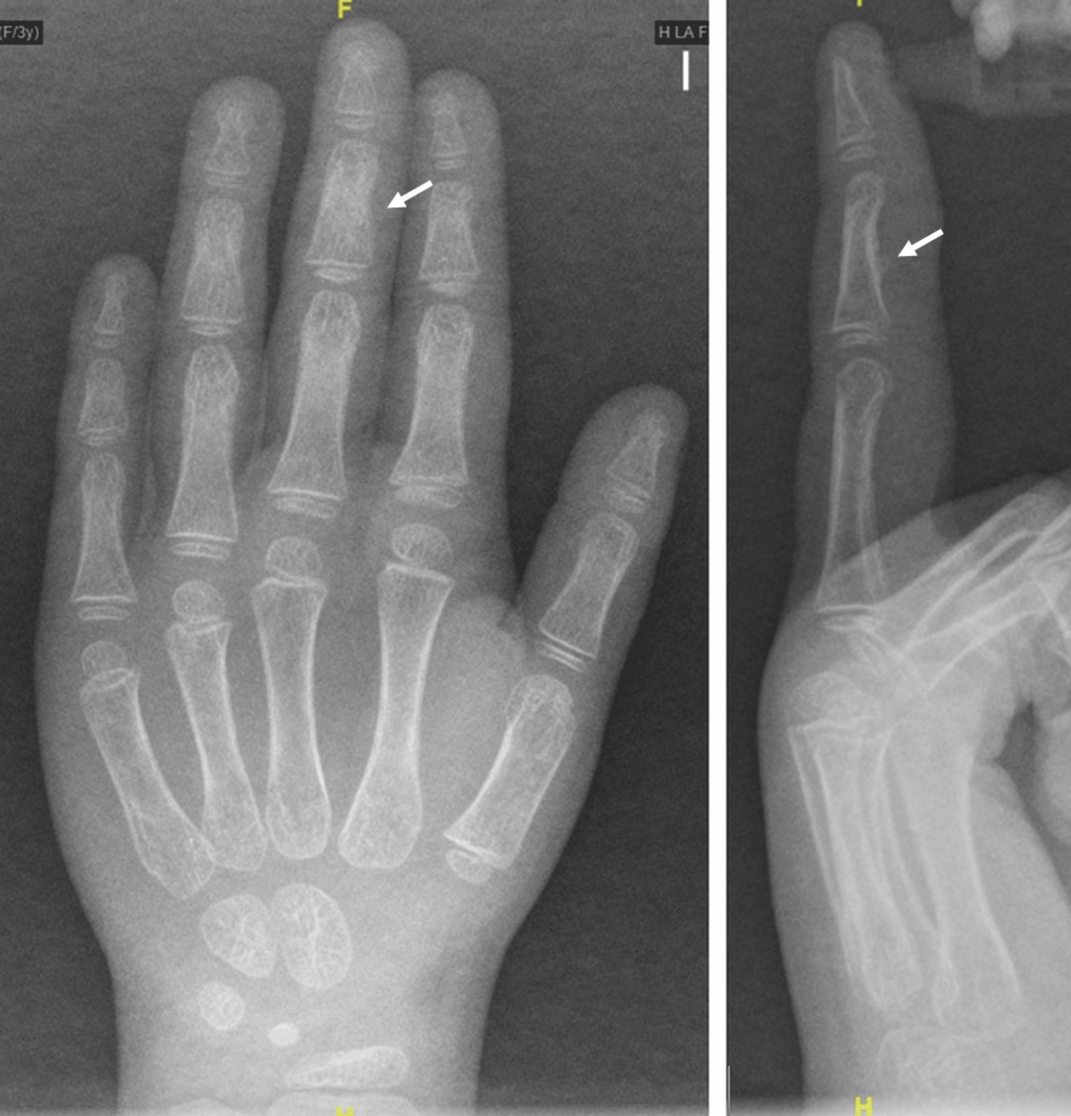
Ossification of the middle phalanx observed on plain radiographs of the hand Left: anteroposterior view; Right: lateral view.
